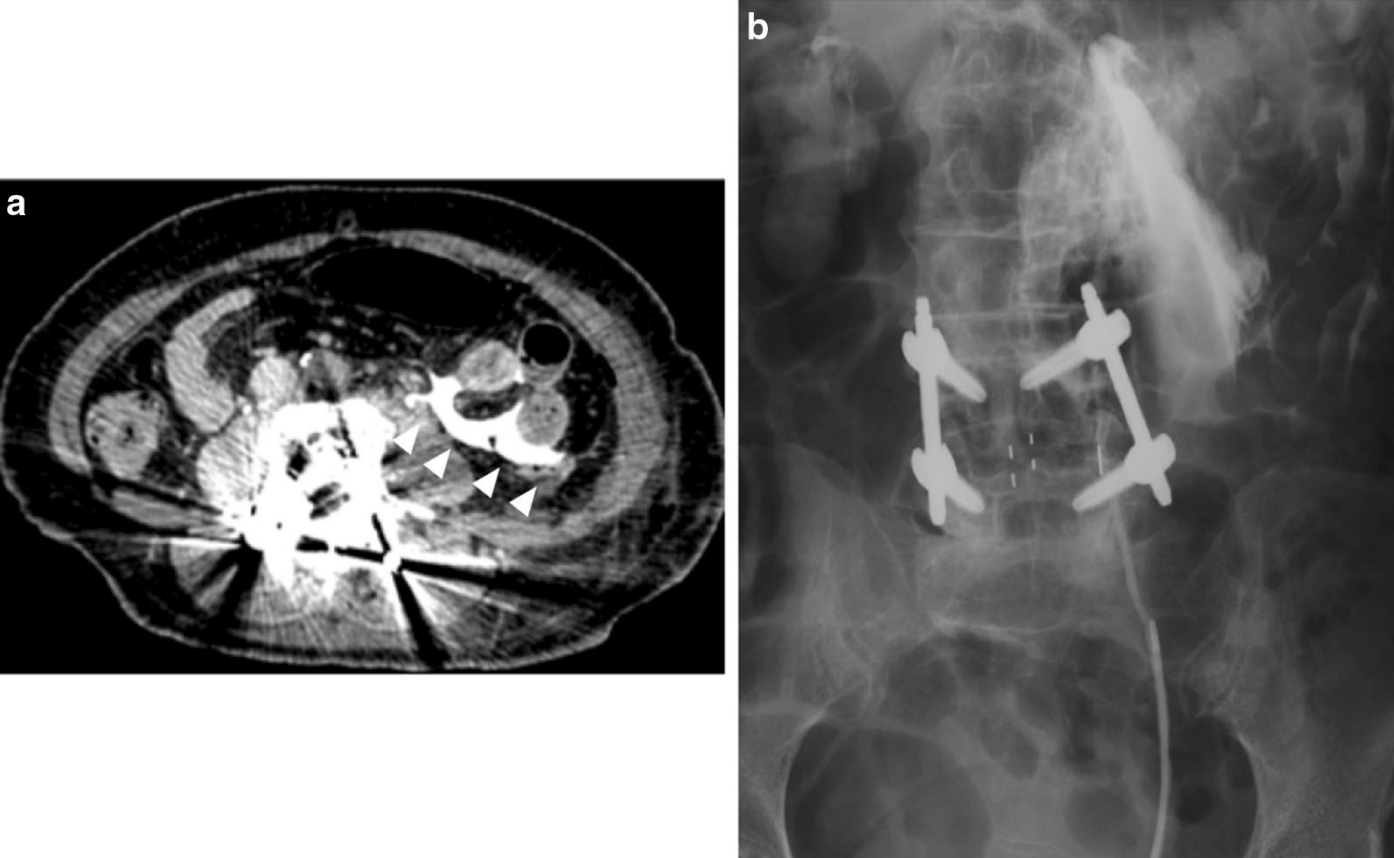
a Delayed-phase enhanced abdominal computed tomography scan at the L4/L5 level shows fluid collection in the left abdominal cavity extending from the left peritoneal space to the posterior iliopsoas region (*arrowheads*). b Left retrograde urography shows extravasation of contrast medium at the L4/5 level
